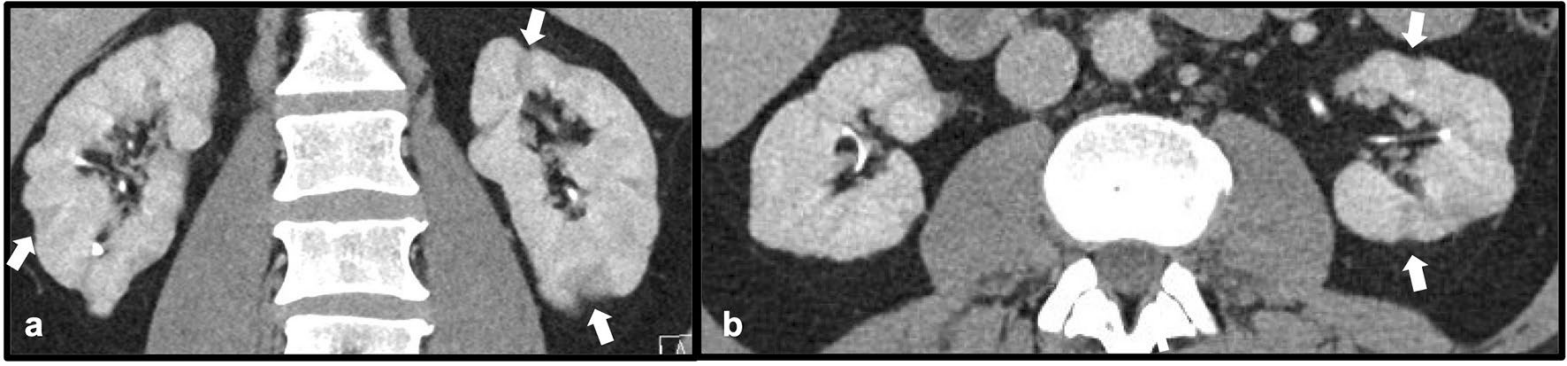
50-year-old male with biopsy-proven cutaneous LCV. Coronal (a) and axial (b) post-contrast CT images demonstrate multifocal peripheral wedge-shaped areas of renal cortical hypoattenuation and parenchymal loss bilaterally (white arrows) consistent with scarring. This patient had normal-appearing kidneys on a PET-CT 7 months prior
